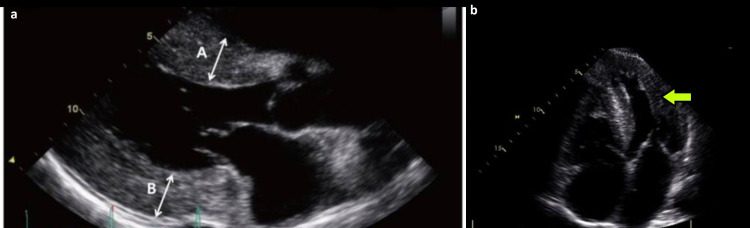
Echocardiography showing left ventricular hypertrophy - parasternal long-axis (a) and apical four chamber (b) view. A: interventricular septum; B: left ventricle posterior wall
